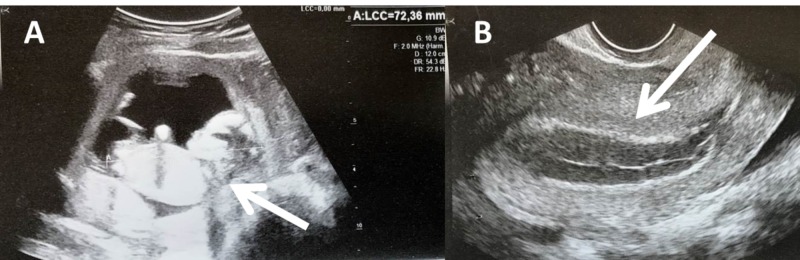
Ultrasound images. (A) First abdominal ultrasound: intrauterine pregnancy (Arrow) with myometrium. (B) Transvaginal ultrasound: vacuity uterine with gravid endometrium (Arrow).
